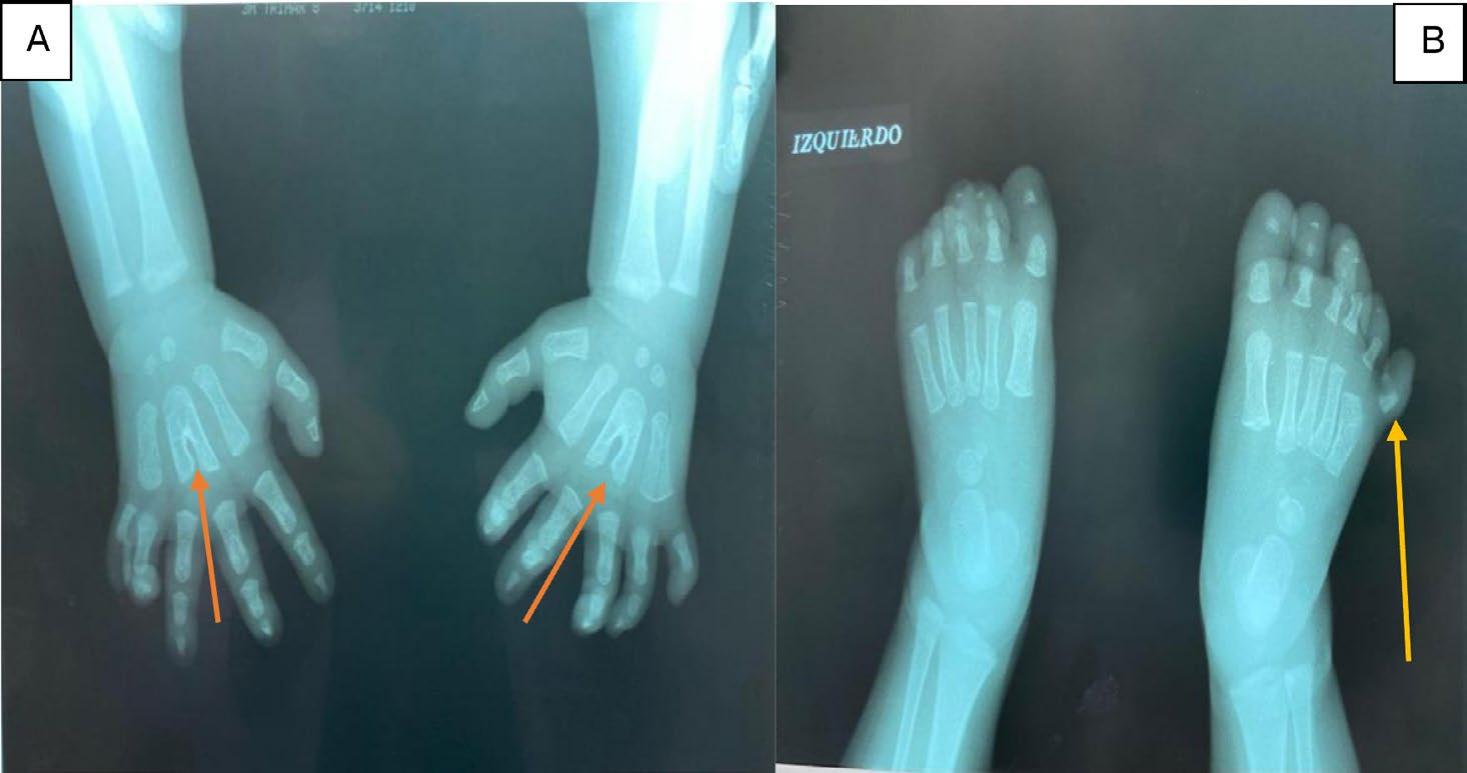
Radiographic images of the hands and feet at 3 months of age, prior to surgery. (A) Hands: Postaxial polydactyly with fusion of the 3rd and 4th metacarpals in a “Y‐shape” in both hands (orange arrow). (B) Feet: Postaxial polydactyly of the right foot (yellow arrow).
